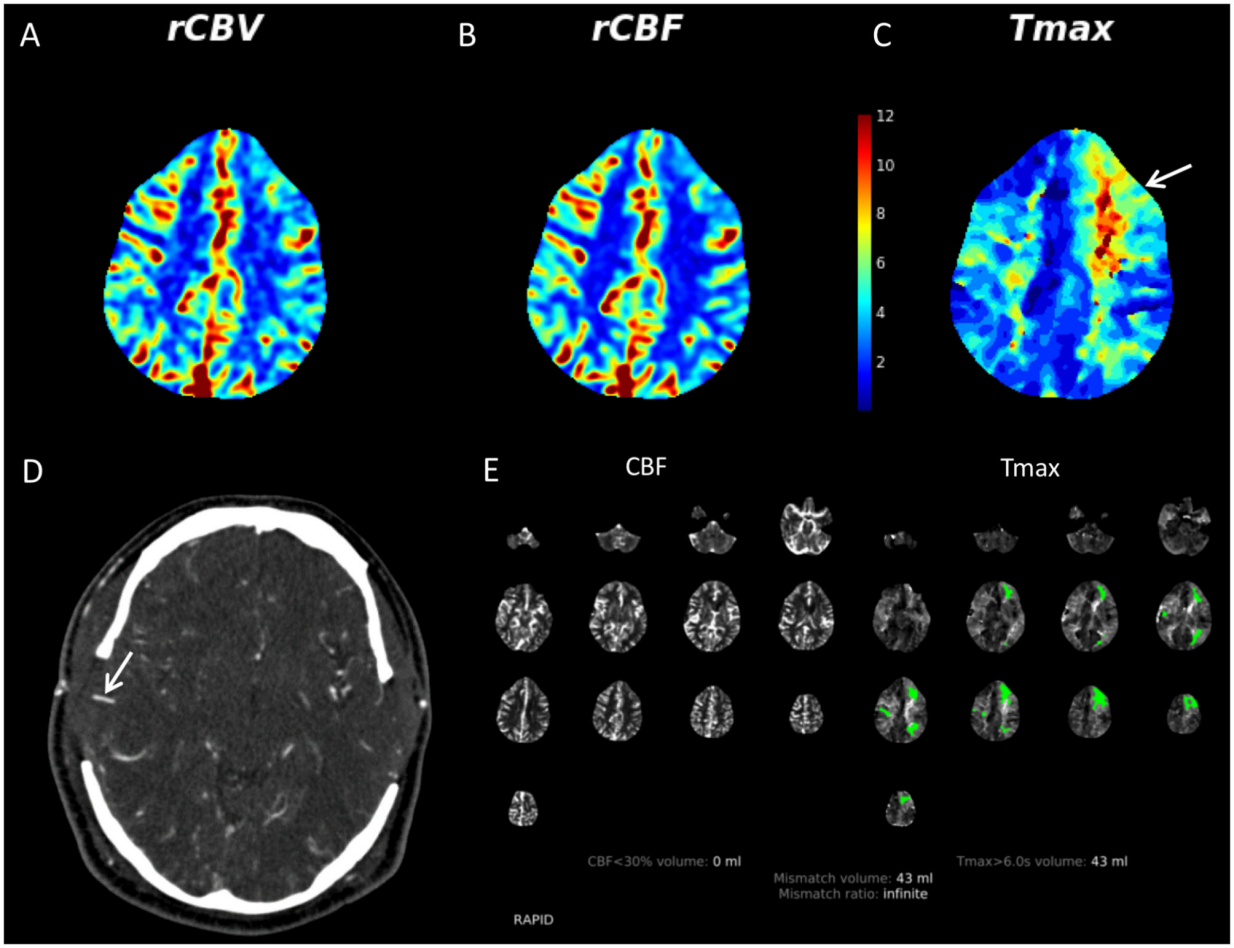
36-year-old male with multiple vascular risk factors presented to our institution with bilateral internal carotid artery terminus narrowing. He had previously undergone a left-sided STA-MCA bypass. He developed aphasia on postoperative day 4 following a right direct STA-MCA bypass (done in conjunction with an indirect right bypass). Given the acuity of the clinical features, the patient was evaluated with CT/CTA/CTP. There was no evidence of acute infarct or hemorrhage on non-contrast CT (not shown). CTP demonstrates mildly increased CBV (A) and CBF (B) in the right MCA territory compared to the left. There is a region of prolonged Tmax in the left frontal lobe (yellow and red colors, white arrow) (C). Tmax, which represents the timepoint at which the residue of the perfusion deconvolution function reaches its maximum value, has been used in recent years to identify areas of brain parenchyma at risk for infarct (so-called penumbra) in the setting of acute stroke. The CTA demonstrates that both direct bypasses are patent (right shown with white arrow) (D). RAPID software (RapidAI, Menlo Park, CA, USA) indicates that there is no region of CBF <30%, suggesting lack of acute infarct (E). Substantial areas of prolonged Tmax (green regions) suggesting ischemic tissue was present. Lack of acute infarct was confirmed on subsequent MRI. This case demonstrates that it is important to have readily available methods to evaluate acute parenchymal, angiographic, and perfusion changes in patients with MMD as part of a MMD program. CBF, cerebral blood flow; CBV, cerebral blood volume; CTP, CT perfusion; MMD, moyamoya disease; STA-MCA, superficial temporal artery to middle cerebral artery.
